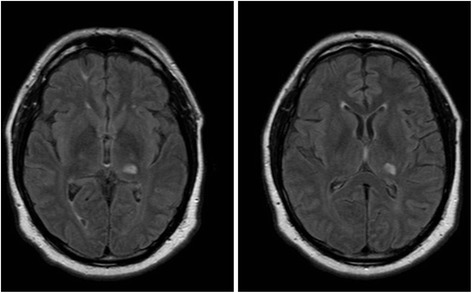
MRI brain, FLAIR sequence, shows infarction of the left posterior thalamus
